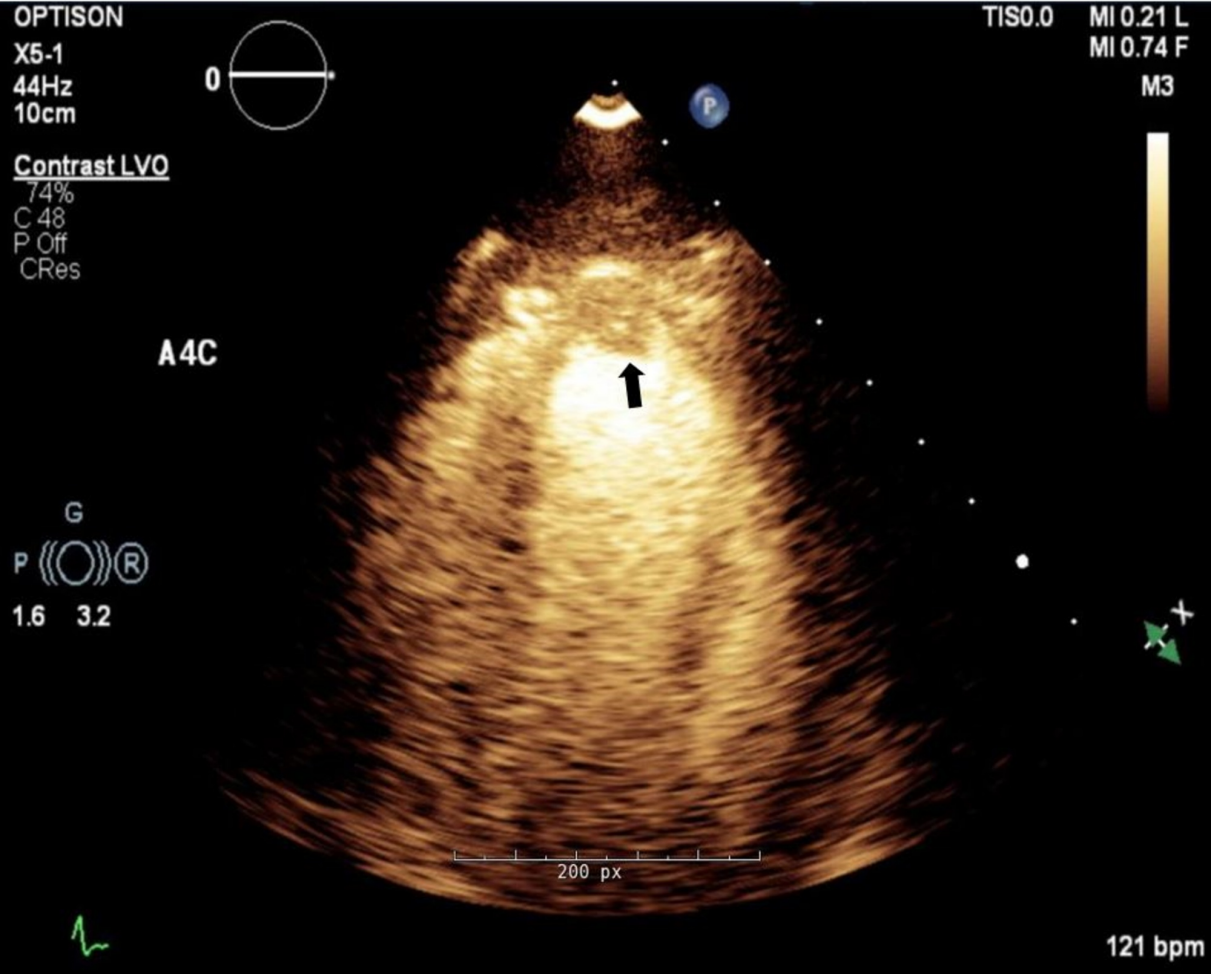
Transthoracic echocardiogram during current hospitalization. Apical four chamber view with commercial contrast demonstrating apical mass concerning for thrombus (black arrow).
